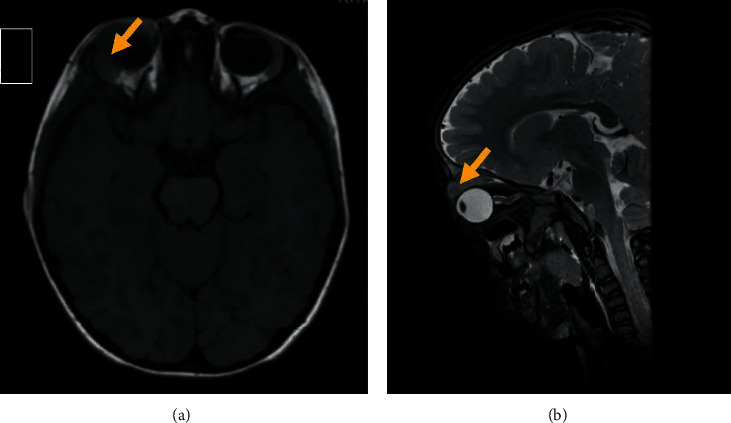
(a, b). Magnetic resonance imaging of the brain for the reported patient. Magnetic resonance imaging of the brain revealed soft tissue T1 and T2 isointense lesion identified in right superior orbit separable from the right lacrimal gland. The lesion appears to be well defined and extraconal. No associated boney erosions or intracranial infiltration was noted. It is indenting and displacing the superior rectus muscle and eye globe. Her optic nerve appeared normal. No brain abnormalities were demonstrated.
